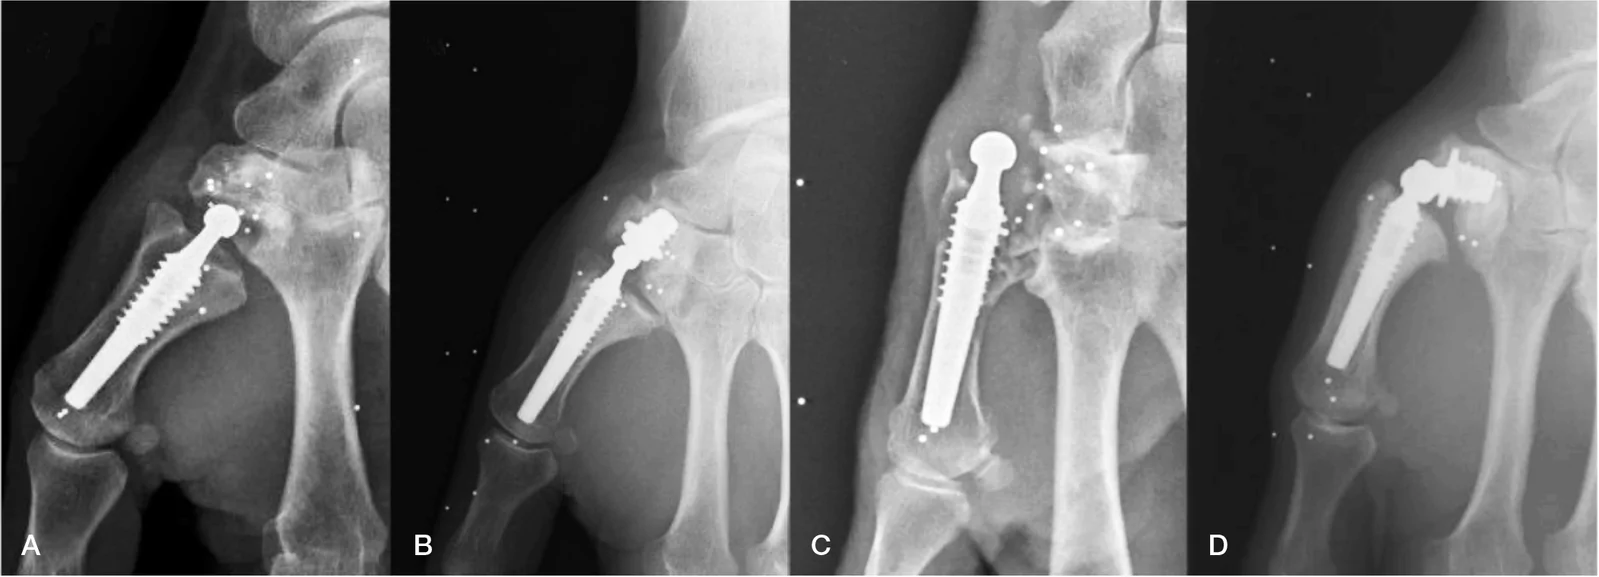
Radiographic implant failures with (A) osteolysis around the proximal stem due to pseudotumor/wear debris, (B) cementless cup with subsidence to the bottom of the trapezium and impingement between the 1st metacarpal and trapezium leading to disconnection of the articulation, (C) dislocation of the head from an all-polyethylene cup, and (D) aseptic cup-loosening, leading to cup tilt in the trapezium bone.
